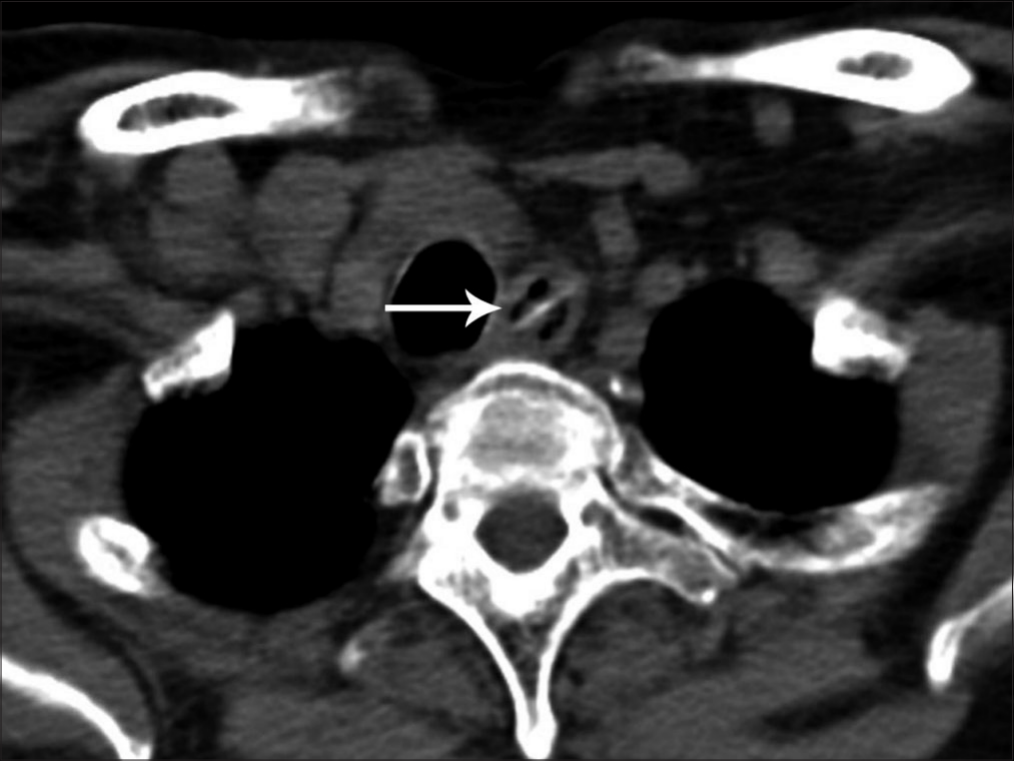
Noncontrast axial CT scan shows a linear radiodense FB (white arrow) in the upper dorsal esophagus. Note that laterally the FB is seen to extend into the wall of the esophagus. On endoscopy, it was found to be partially embedded within the wall of esophagus, with the presence of a small tear
